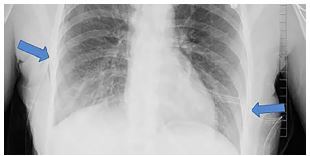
Chest radiograph following bilateral chest tube placement for bilateral pneumothoraces. Arrows mark chest tube insertion sites.
